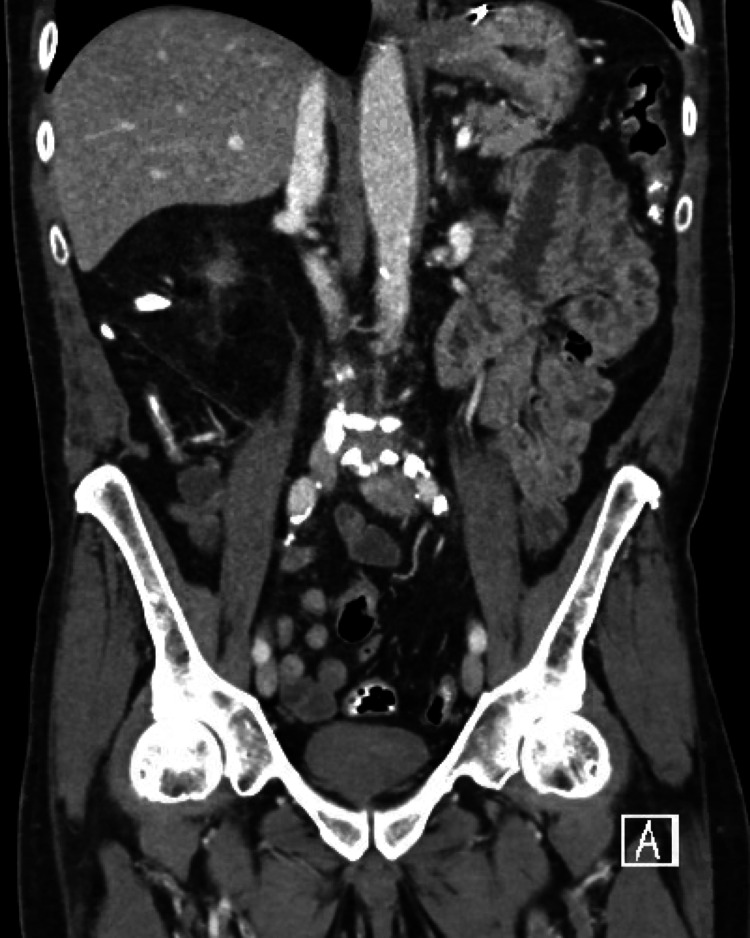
Previous CT scan without any evidence of colitis
